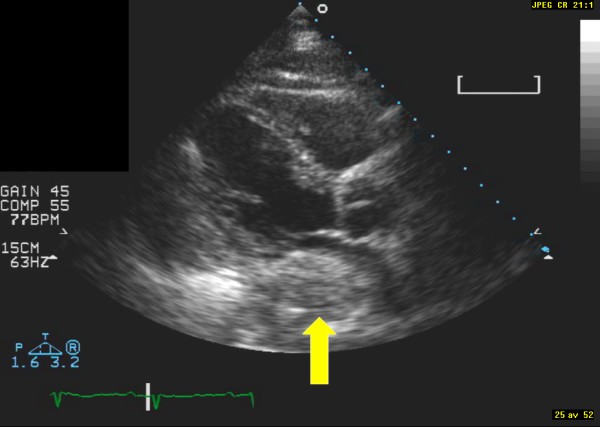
Image from parasternal long-axis view. The arrow indicates the hiatal hernia.
